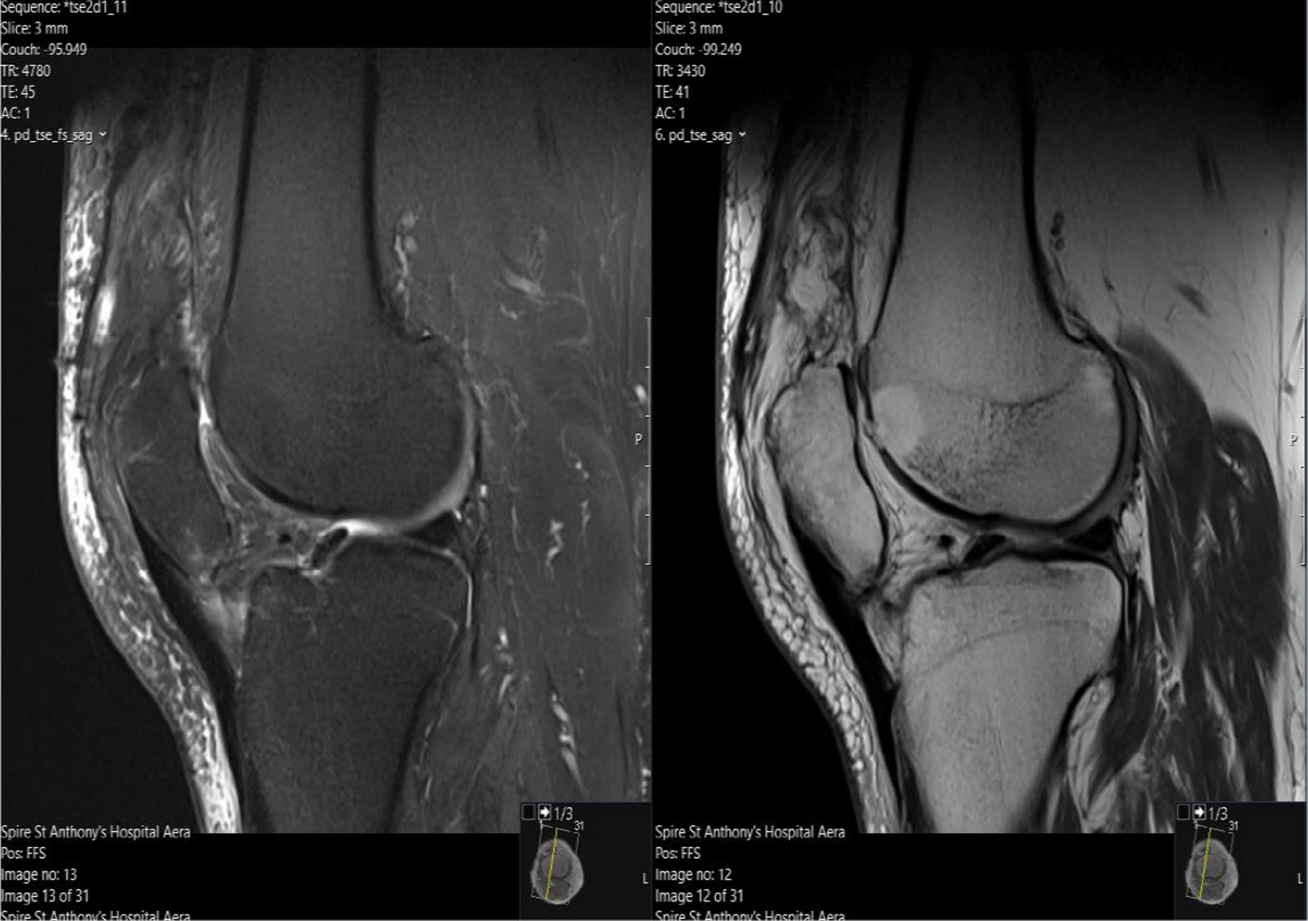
T1 and T2-weighted Magnetic Resonance Imaging  showing chronic right knee quadriceps tendon rupture, with tendon retraction, scarring, and fluid signal
